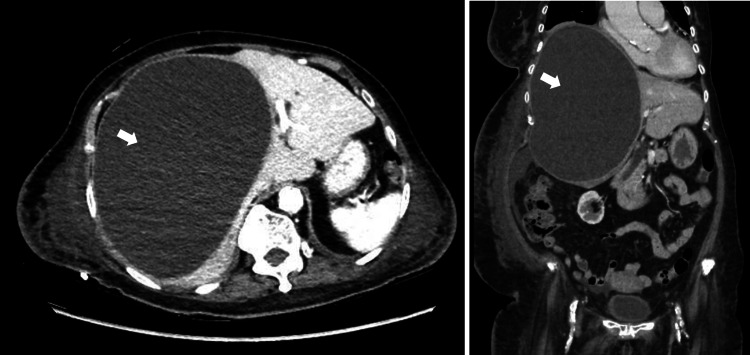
CT scan of abdomen and pelvis (transverse and coronal views) revealing a polycystic liver with a large hepatic cyst measuring 18.9 cm x 13.6 cm x 21.0 cm (white arrows).
